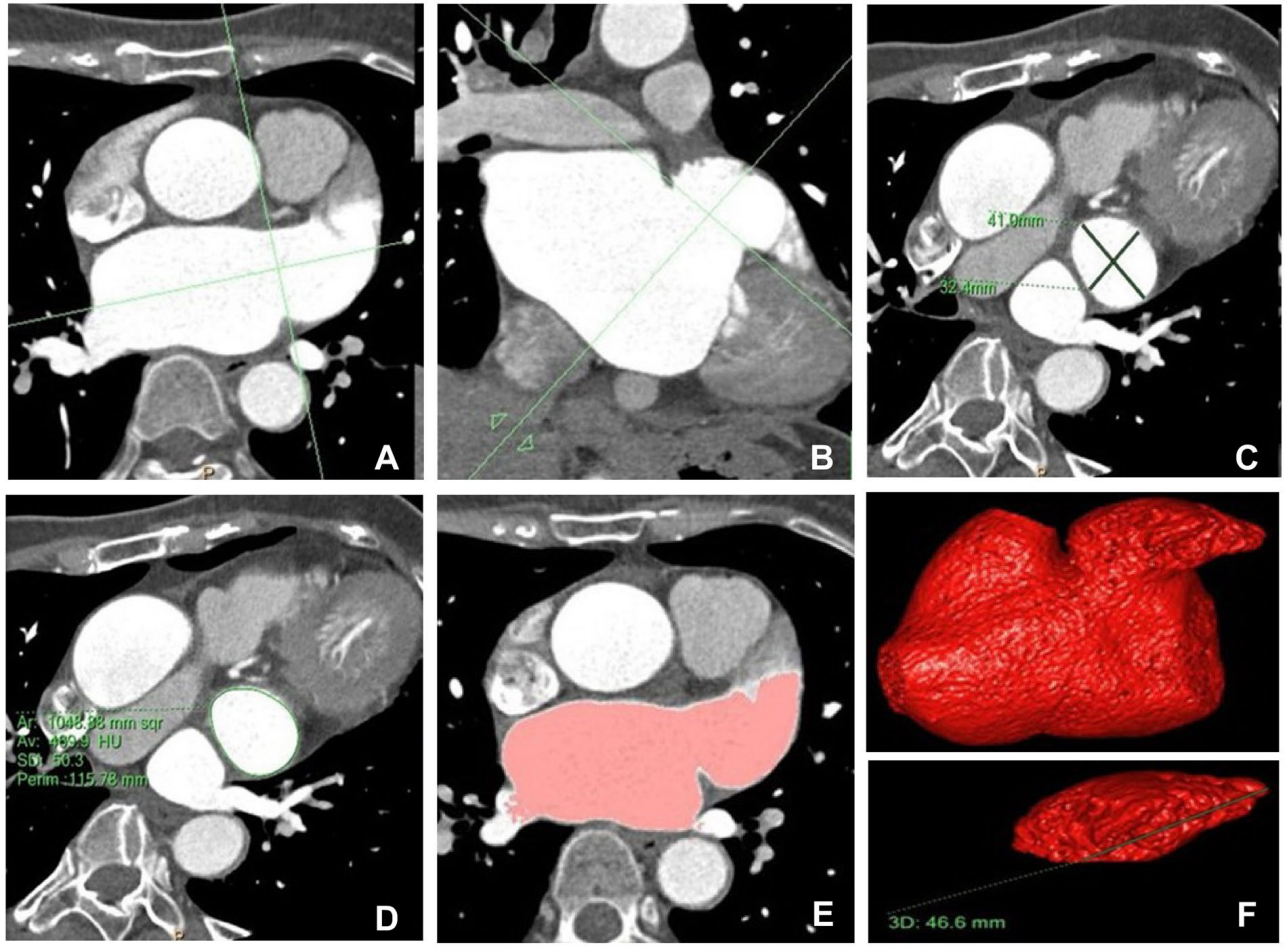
Quantitative measurement of left atrium and left atrial appendage (LAA). (A–C) In computed tomographic imaging, the coronary positioning line was put across the conjunction of the left atrium with LAA in the cross section (A,B) to obtain the cross section of the orifice of the LAA (C). The major and minor axes of the LAA were measured (C). (D) The perimeter of the LAA orifice was measured. (E,F) The left atrium and LAA imaging was obtained using cardiac function software for measuring the volume of the left atrium and LAA. The LAA depth was measured (F, lower part) from the most distal tip of LAA to the center of the cross section of the LAA orifice. The upper part in F is the left atrium and LAA.
